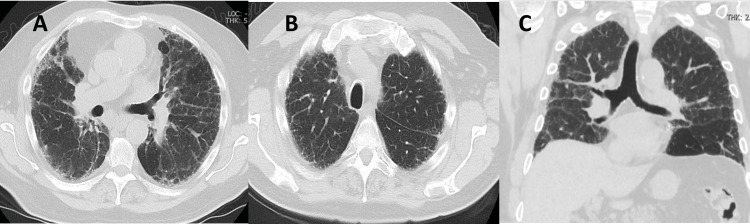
CT scan showing resolution of ground glass opacities. A, transverse view of lung (proximal); B, transverse view of lung (distal); C, sagittal view of lung
